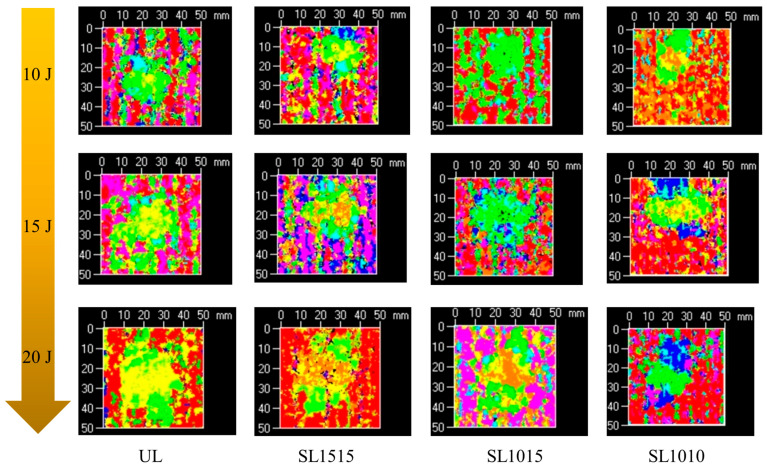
Ultrasonic C-scan images of groups UL, SL1515, SL1015 and SL1010 subjected to impact energies of 10 J, 15 J, and 20 J.
